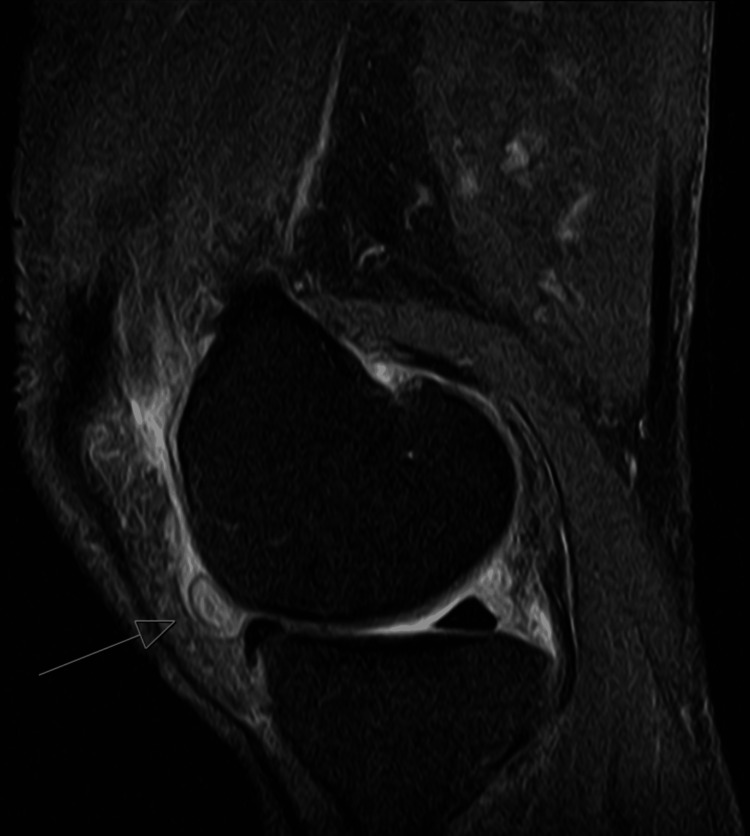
MRI of right knee obtained one month after completion of initial course of antifungal therapy showing mass-like synovial thickening, a sequela of Histoplasma capsulatum septic arthritis.
